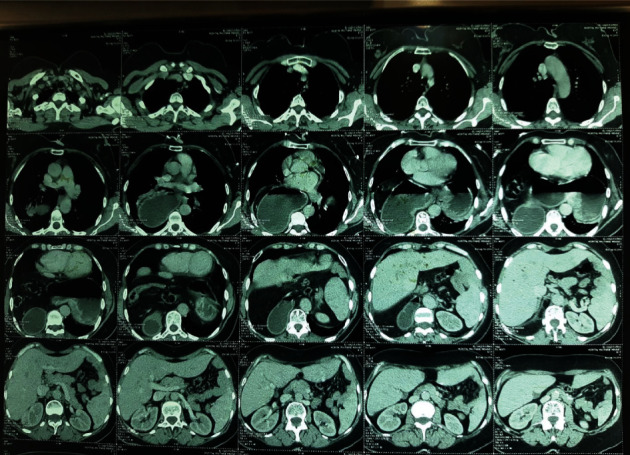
A thoracoabdominal CT scan showing a stomach stasis on a pyloric obstacle.
